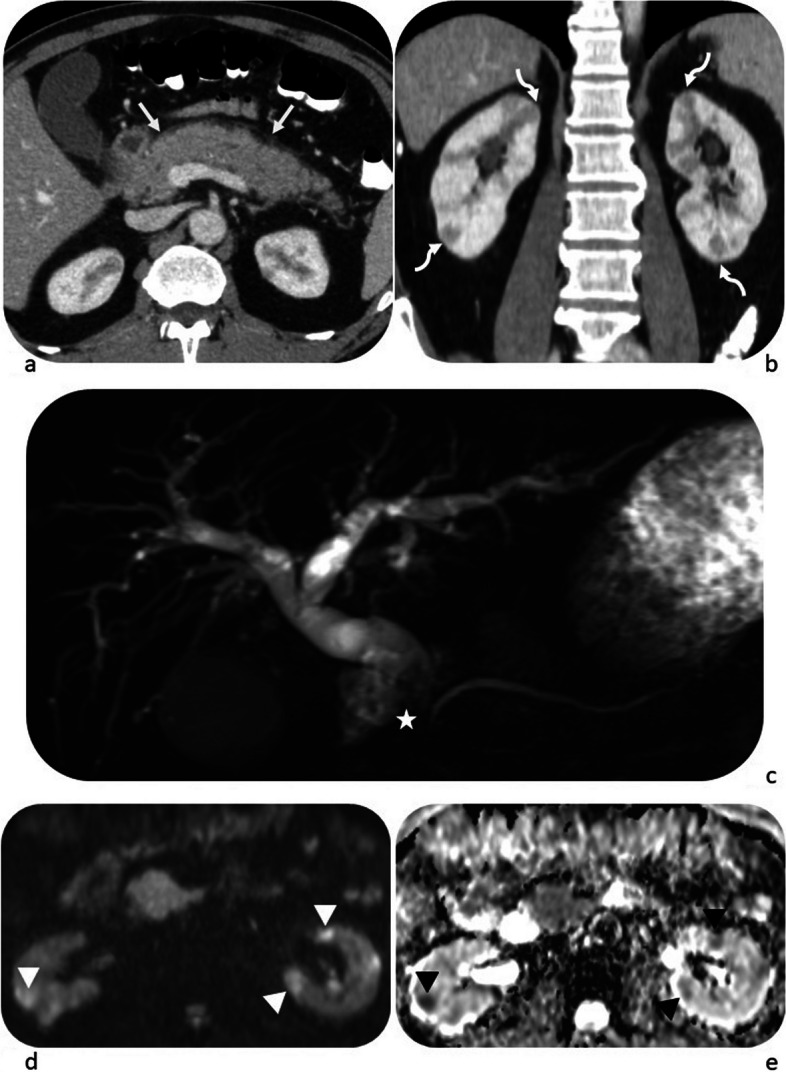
Case of pancreaticobiliary involvement scoring 19 points, bilateral renal involvement scoring 10 points and a 5-fold increase above the normal limit for serum IgG_4_ scoring 11 points. Overall score = 40 points. a Axial portal venous phase CT image shows diffuse pancreatic enlargement, with peripancreatic stranding in a hypoattenuating rind-like pattern/pseudocapsule (white arrows). b Coronal reformat portal venous CT image shows multiple bilateral nodular and wedge-shaped hypoattenuating lesions (curved arrows). c Coronal MRCP image shows dilation of the intra- and extra-hepatic bile ducts down to a smooth narrowing of the distal CBD over 2-cm segment (white star). The pancreatic duct is smooth and of a normal calibre. d, e Axial DWI & ADC map images show multifocal areas of restricted diffusion in both kidneys (arrowheads)
